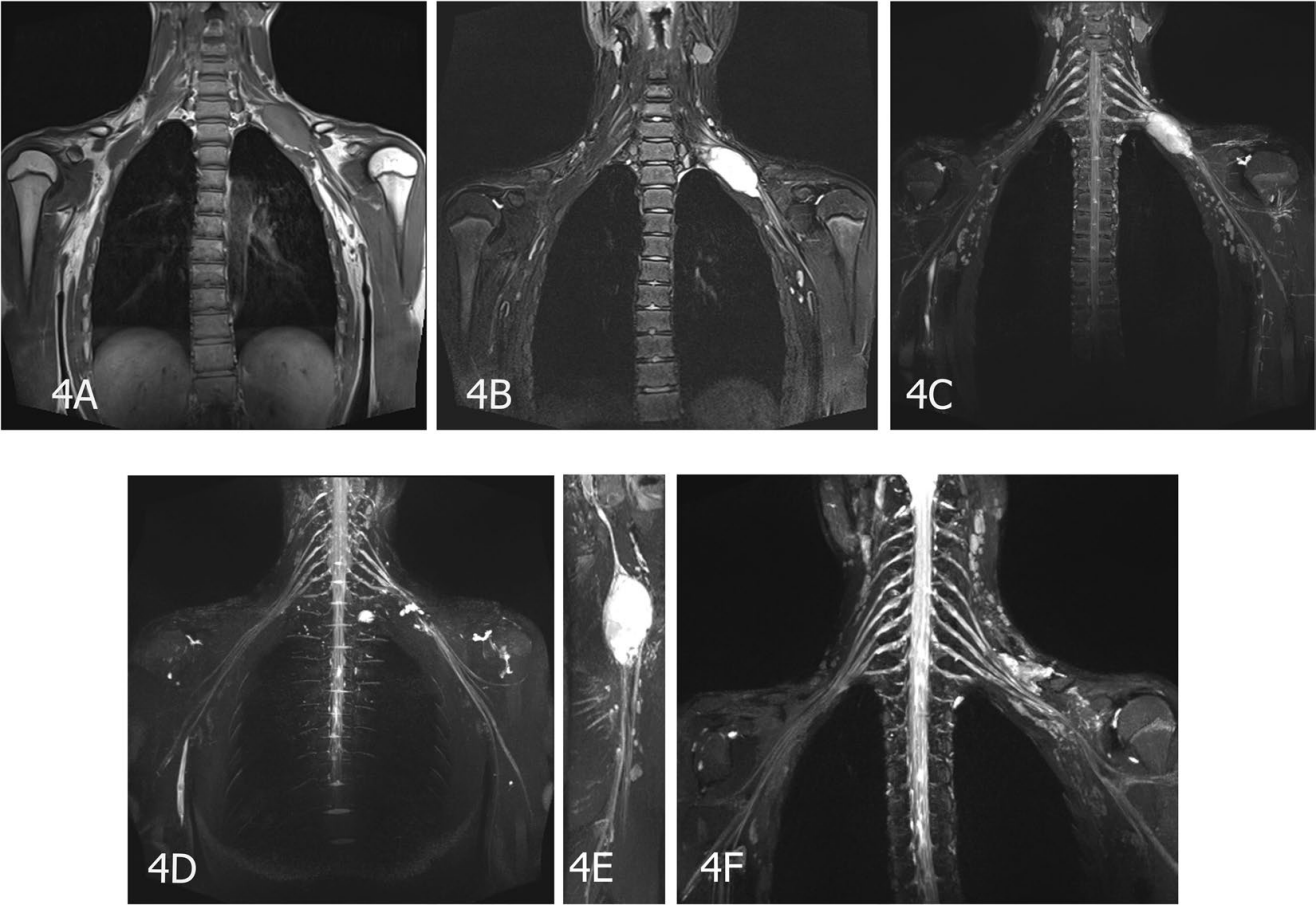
A 15-year-old male patient with an MPNST in the left C5 spinal nerve. (A) Coronal T1-weighted images (T1WI), isointense signal with ill-defined mass located in the left supraclavicular region. (B) On coronal T2-weighted fat-suppressed imaging image (T2WI-FS), the lesion shows hyperintense signal with well-defined shape. (C–E) 3D-STIR SPACE and enhanced images show that the tumor was originated from the left brachial plexus. (F) Enhanced 3D-STIR SPACE image was received after postoperative examination.
